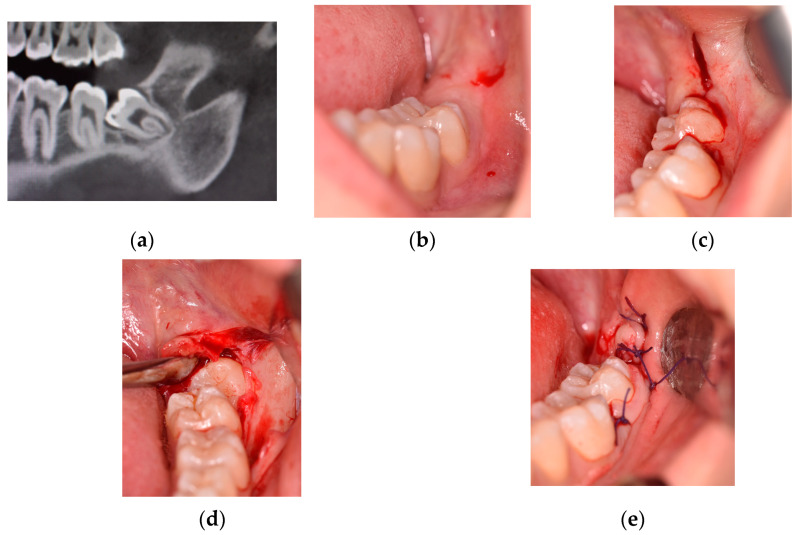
Flap with papilla detachment (class IC position): (a) pre-operative detail of cone beam TC evaluation; (b) pre-operative clinical evaluation; (c) intra-operative distal-releasing incision; (d) intra-operative detachment of gingival papilla between the first and second molars; and (e) final wound closure.
